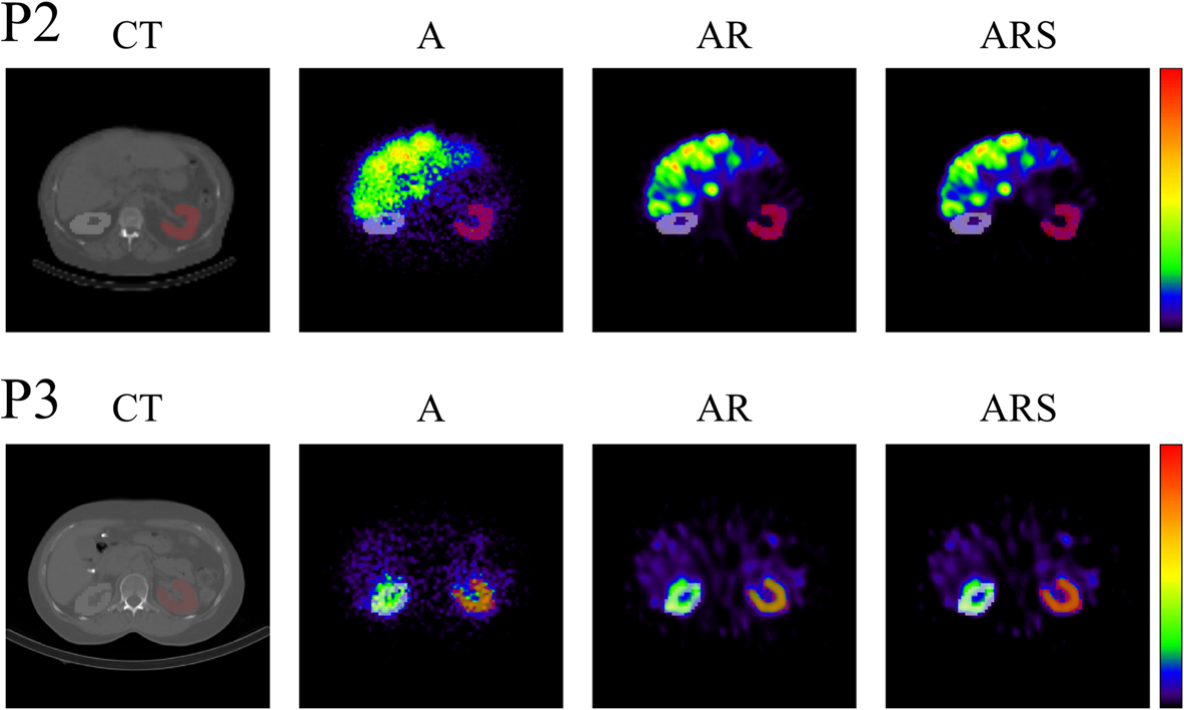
Representative transverse SPECT/CT slices reconstructed using A, AR and ARS compensation methods of P2 and P3 subjects treated with ^177^Lu-DOTATATE
